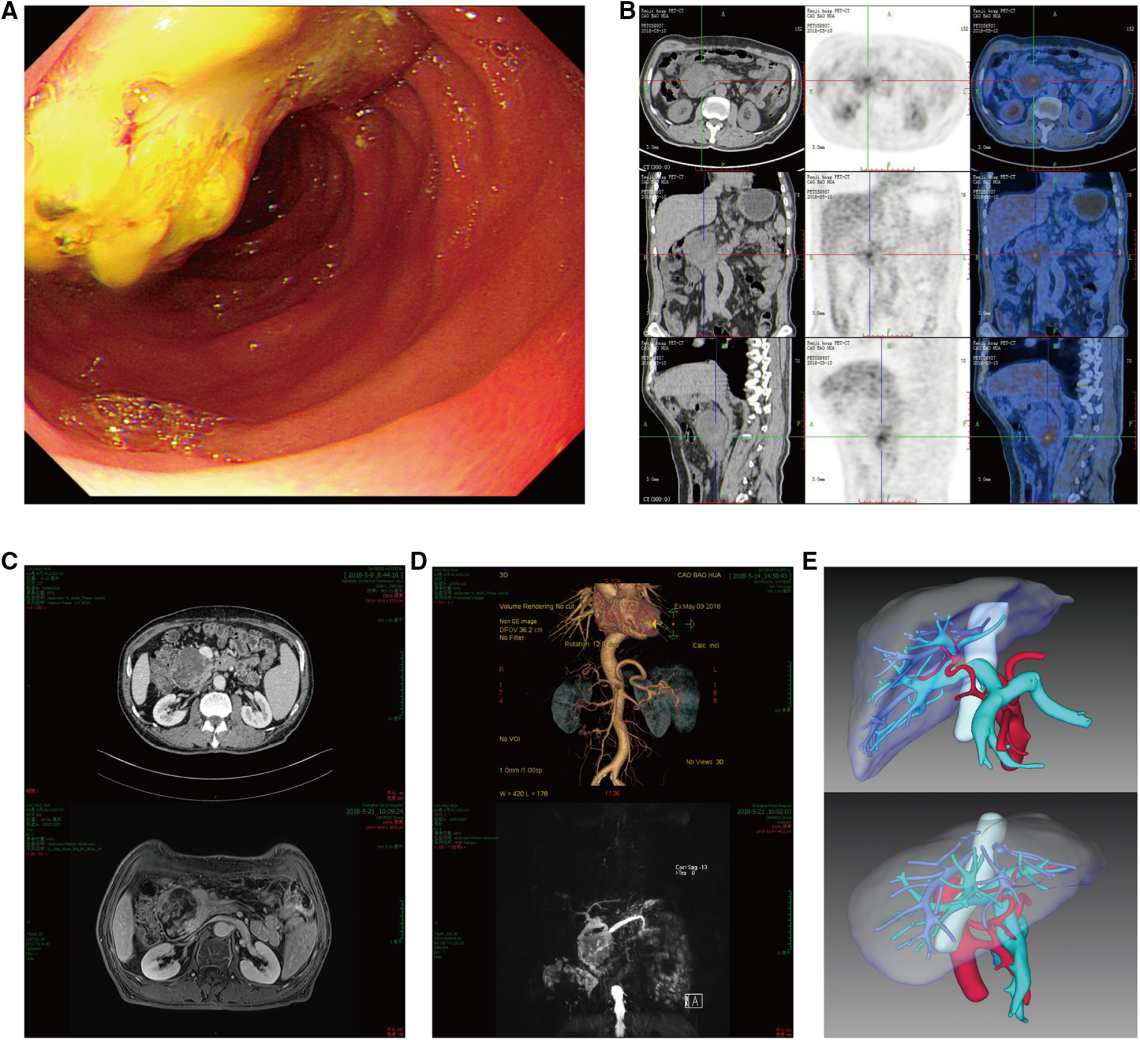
(A) gastroduodenoscopy revealed a mass lesion located in the descending part of the duodenum. (B) PET-CT scan showed a tumor in the duodenum with SUVmax = 5.5, its size was about 6.4 × 6.7 cm. (C) Contrast-enhanced computed tomography (CT) and magnetic resonance imaging (MRI) detected a tumor of approximately 6.5 cm in diameter that was located in duodenum. (D) Magnetic resonance cholangiopancreatography (MRCP) found that duodenal tumor caused dilation of extrahepatic bile duct and pancreatic duct. (E) CT angiography and three-dimensional reconstruction revealed donor liver's artery went from the back down side of portal vein to the front right side of it, the gastroduodenal artery is visible.
